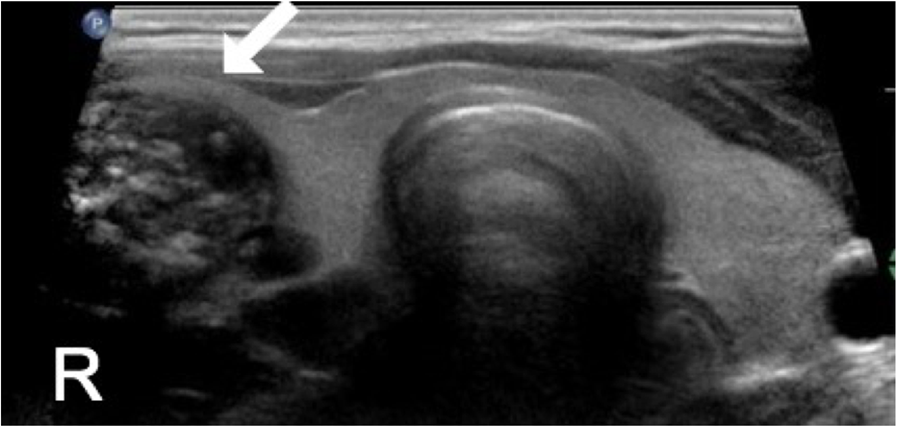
Second ultrasound. Transverse view of the thyroid ultrasound showing right lobe, isthmus and left lobe. The isthmus and the left lobe are noted for homogenous echotexture. Right lobe (white arrow) is enlarged due to multiple nodules
